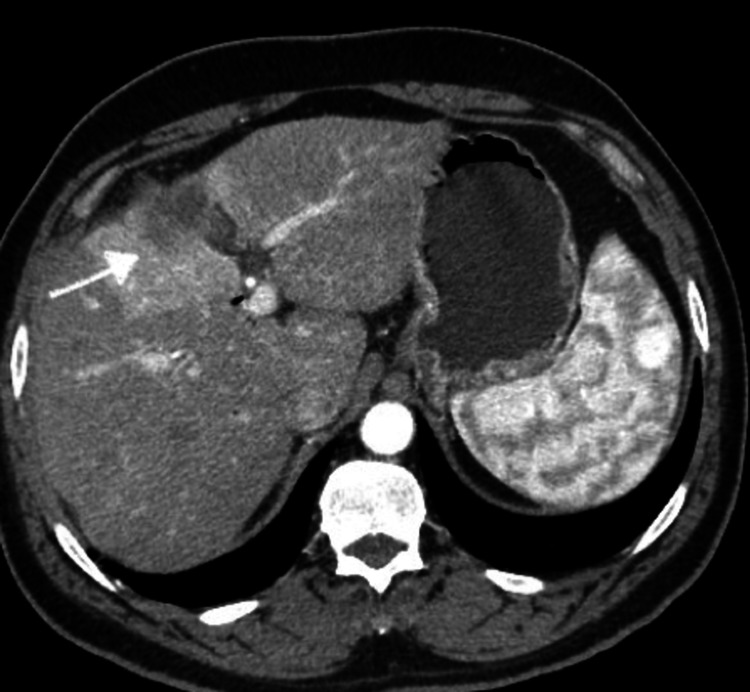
CT abdomen (Case 1) The figure indicates disease stability post 14 months of second-line gemcitabine/nab-paclitaxel.
